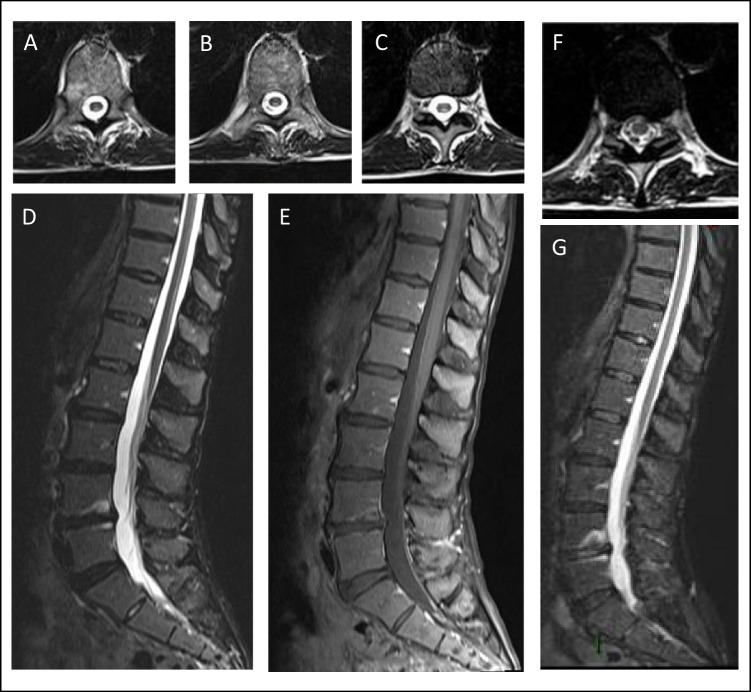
Magnetic resonance imaging: extensive hyperintensity on T2-weighted (A, B, C) and T2-TIRM (D) sequences in the dorsal columns of the lower thoracic spinal cord, 10 months after symptoms onset. After gadolinium injection, no contrast enhancement was detected (E). One year later, signal abnormalities were no longer visible despite the persistence of clinically significant sensory deficits (F, G)
